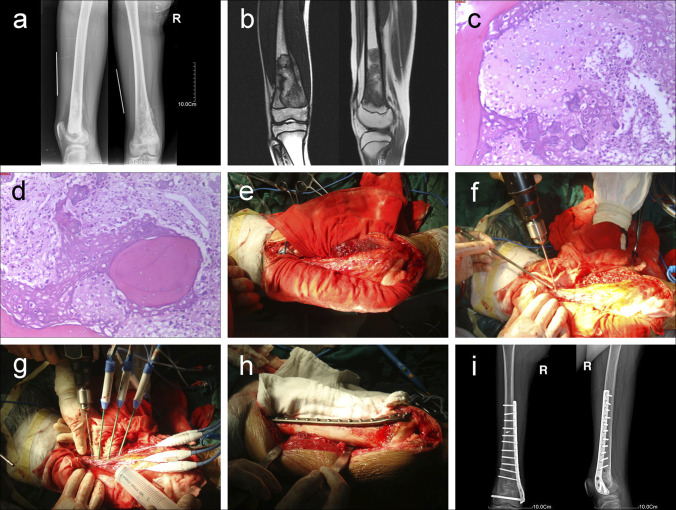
A–I, (A) AP and lateral radiographs and (B) MRI showing an osteosarcoma of the distal femur. C and D, Pathologic examination results of preoperative biopsy (E) separating the tumor-bearing segment from surrounding normal tissues and placing wet gauze between the tumor bone and surrounding normal tissues. F and G, Setting up the antenna array and performing en bloc MW ablation of the tumor segment, (H) the bone defect was filled with mixture of bone cement and bone graft, and prophylactic internal fixation was performed to strengthen the devitalized bone. I, Postoperative AP and lateral radiographs.
